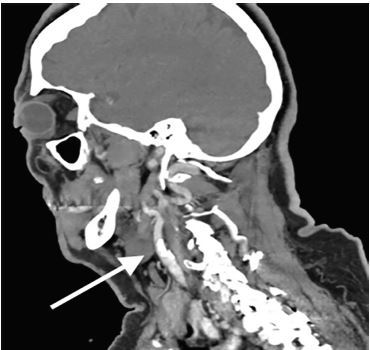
Sagittal view of computed tomography with angiography of the head and neck identifying an intimal flap in the left internal carotid artery consistent with a carotid artery dissection.
